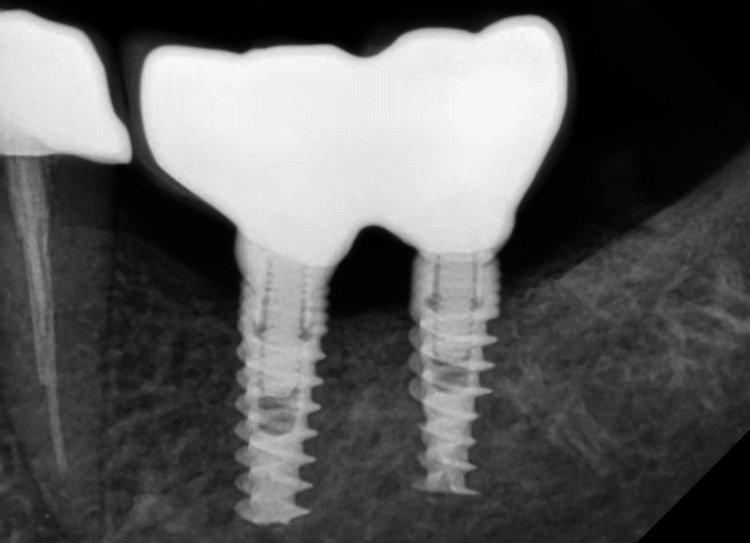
Postoperative IOPA IOPA: intraoral periapical radiograph
